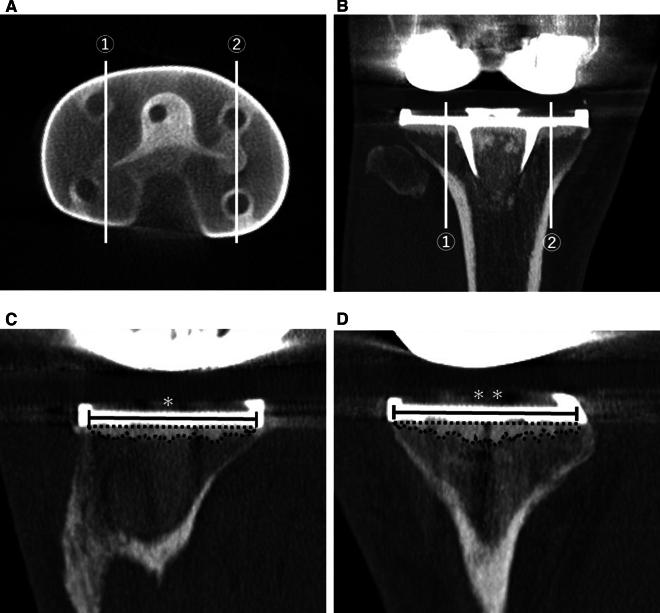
Evaluation method of mean cement penetration on sagittal section in postoperative computed tomography (CT) image. A Postoperative CT axial section just under the tibial baseplate. B Postoperative CT coronal section at the most posterior level of the fin. In (A) and (B), the (1) lateral side of sagittal section and (2) the medial side of sagittal section level are shown. The level of the sagittal section was one slice lateral to the section in which the most posterior portion of the fin of the tibial component was delineated for the medial and lateral sides. C Postoperative CT sagittal section of the lateral side. D Postoperative CT sagittal section of the medial side. In (C) and (D), the area surrounded by the black dotted line represents the cement penetration area (mm^2^). The single and double asterisks indicates the base of the lateral side (mm) and the base of the medial side (mm), respectively. Mean penetration: area/base (mm)
